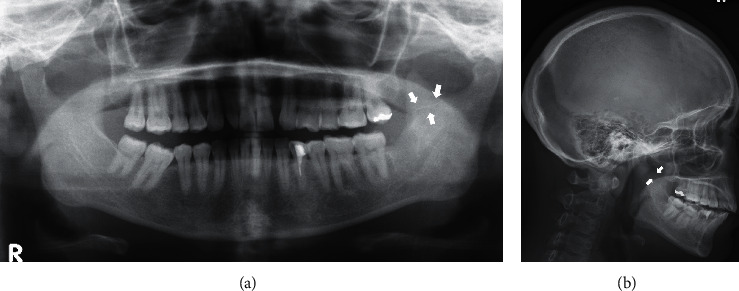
(a) Panoramic X-ray performed at the first visit. The broken injection needle can be seen at the base of the left coronoid process (arrows). (b) Lateral cephalometric X-ray. The broken injection needle can be seen at the base of the left coronoid process (arrows).
